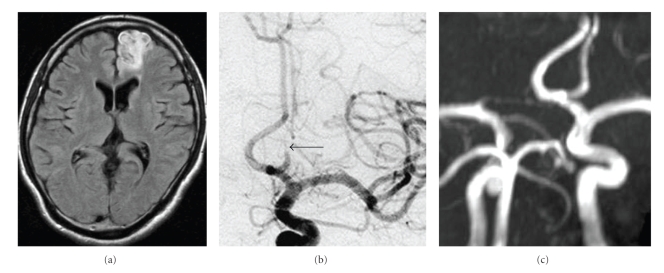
(a) Magnetic resonance imaging (FLAIR sequence) about one month after the onset shows infarction of the medial part of the left frontal lobe. (b) Left internal cerebral artery angiogram displays severe stenosis of the A_2_ segment (arrow). (c) About 9 months after the onset, magnetic resonance angiography reveals recanalization of the left A_2_ segment.
